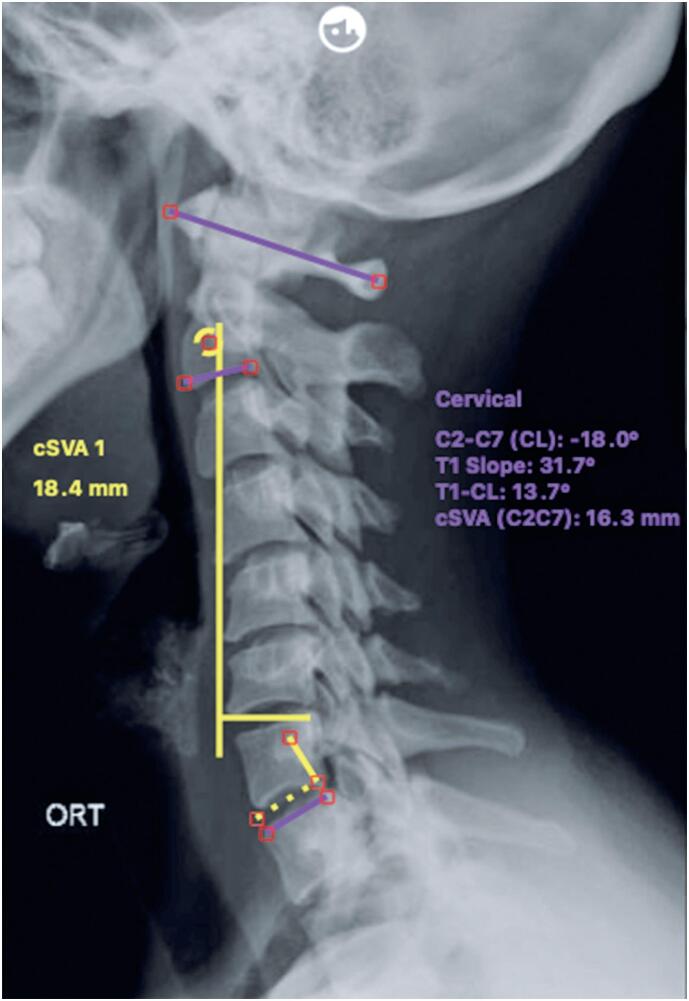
Cervical sagittal alignment parameter measurements as performed on radiographs using Surgimap software
